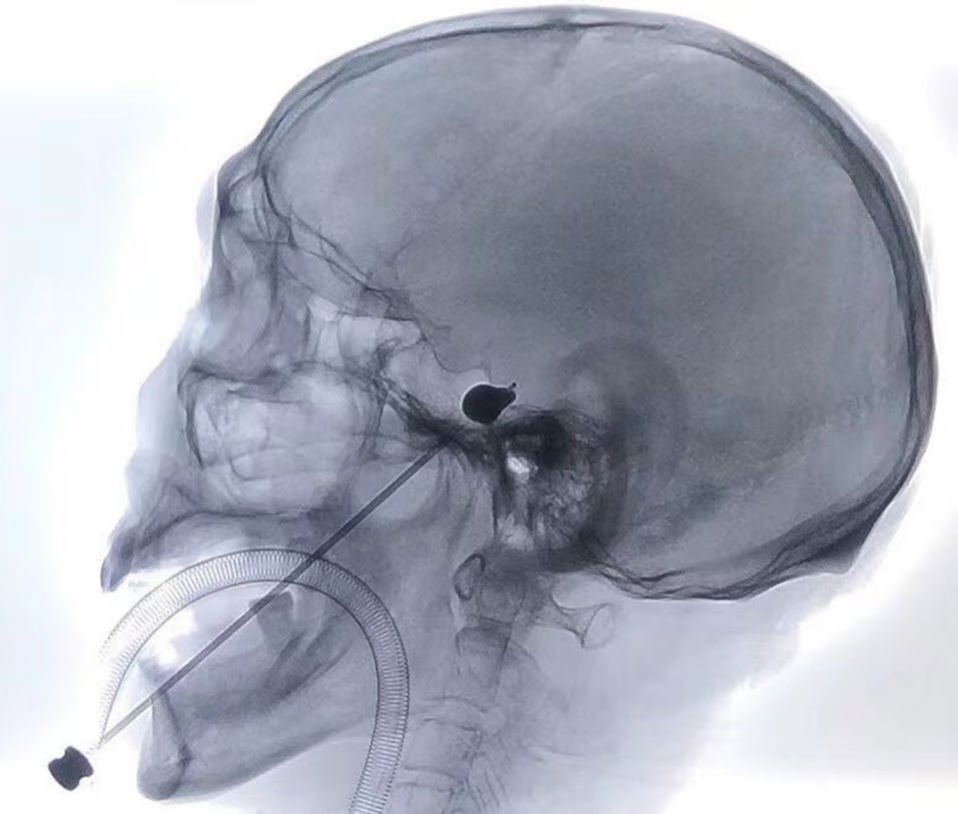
The ideal pear-shaped balloon was confirmed on lateral radiography
